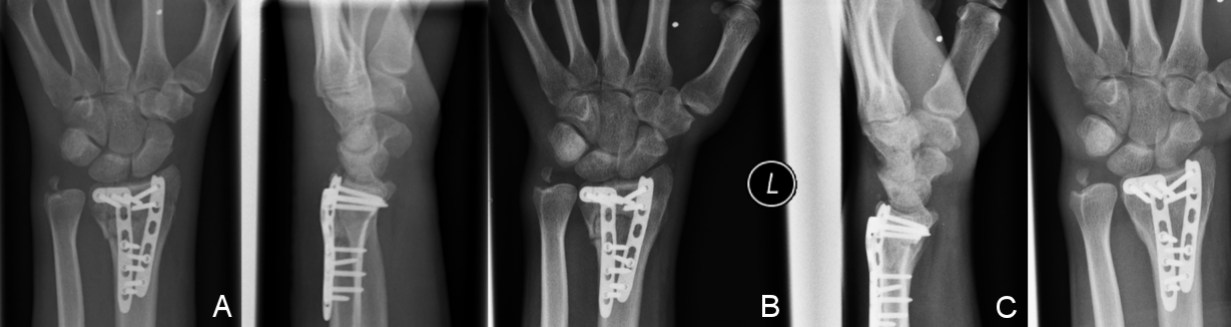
Radiographic follow-up series of a 46-year-old female patient with an AO 23C3 fracture, treated through a dorsal approach with 2.4 mm LCP (Synthes^®^). A) Postoperative x-ray with excellent reduction. B) Follow-up radiographs after 12 weeks. C) Follow-up radiograph one year after trauma with excellent radiological fracture consolidation and no significant loss of reduction.
